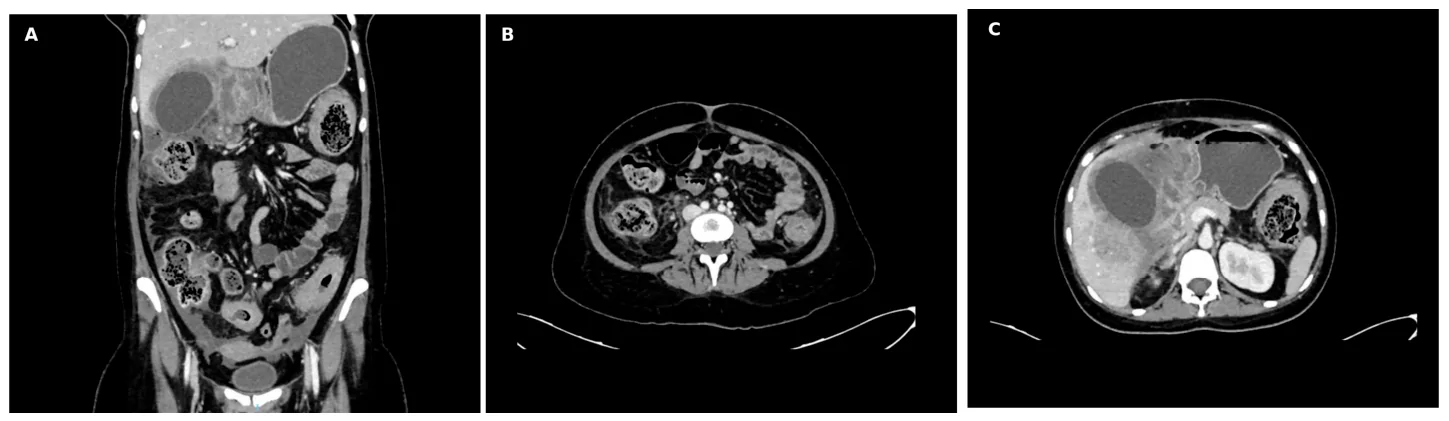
Repeat CT after cholecystectomy. (A) Coronal reformat showing a loculated collection in the gallbladder fossa and a distended, fluid-filled stomach reflecting gastric outlet obstruction; (B) axial image showing persistent multisegmental colonic wall thickening; (C) axial image of the upper abdomen showing the loculated subhepatic collection at the gallbladder bed.
